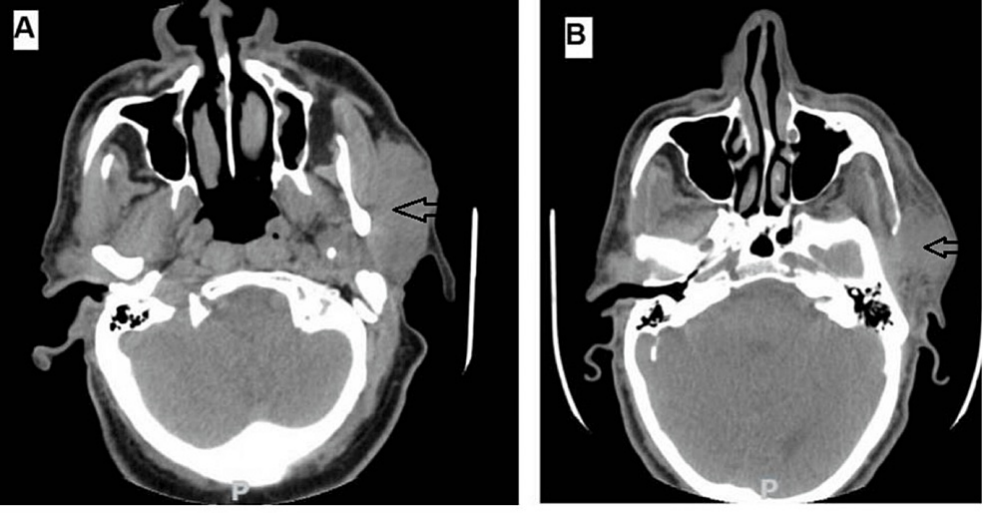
CT showing the masses (arrows) in the parotid gland. (A) Primary lesion. (B) Recurrent lesion. CT, computed tomography
